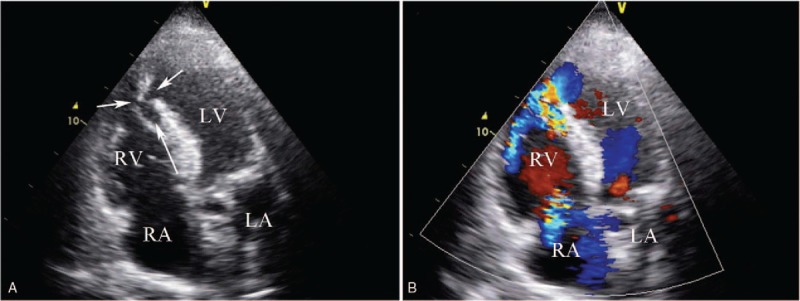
(Patient #12) Transthoracic echocardiogram (apical 4 chamber views) showing interventricular septal dissection following an anterior septal myocardial infarction. (A) The short arrows indicate the rupture of the interventricular septum both in left ventricle and right ventricle side. (B) Color flow (long arrow) shows the dissecting cavity of interventricular septum. LA = left atrium, LV = left ventricle, RA = right atrium, RV = right ventricle.
